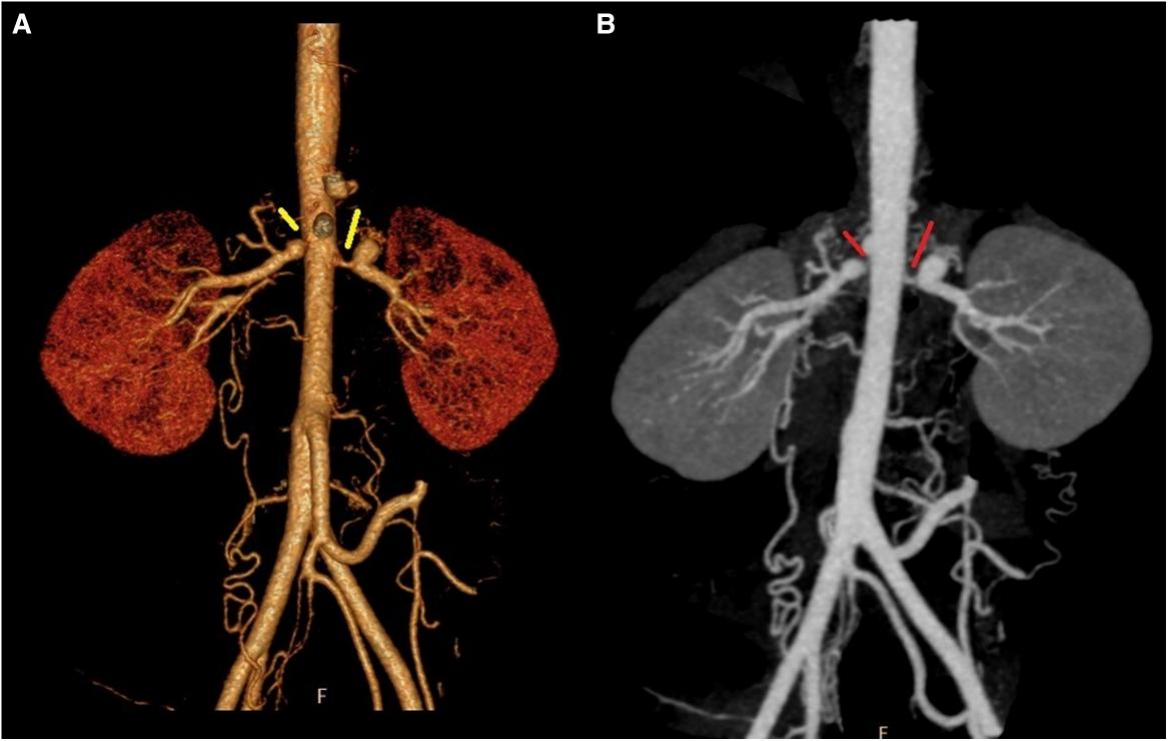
Computed tomography angiography. *A*, *B*) Coronal plane showing the right and left renal artery aneurysm with tight osteal stenosis. Computed tomography Abdomen reveals severe osteoproximal left renal artery stenosis with aneurysm formation. Moderate to severe stenosis of right ostioproximal stenosis with small aneurysm formation.
